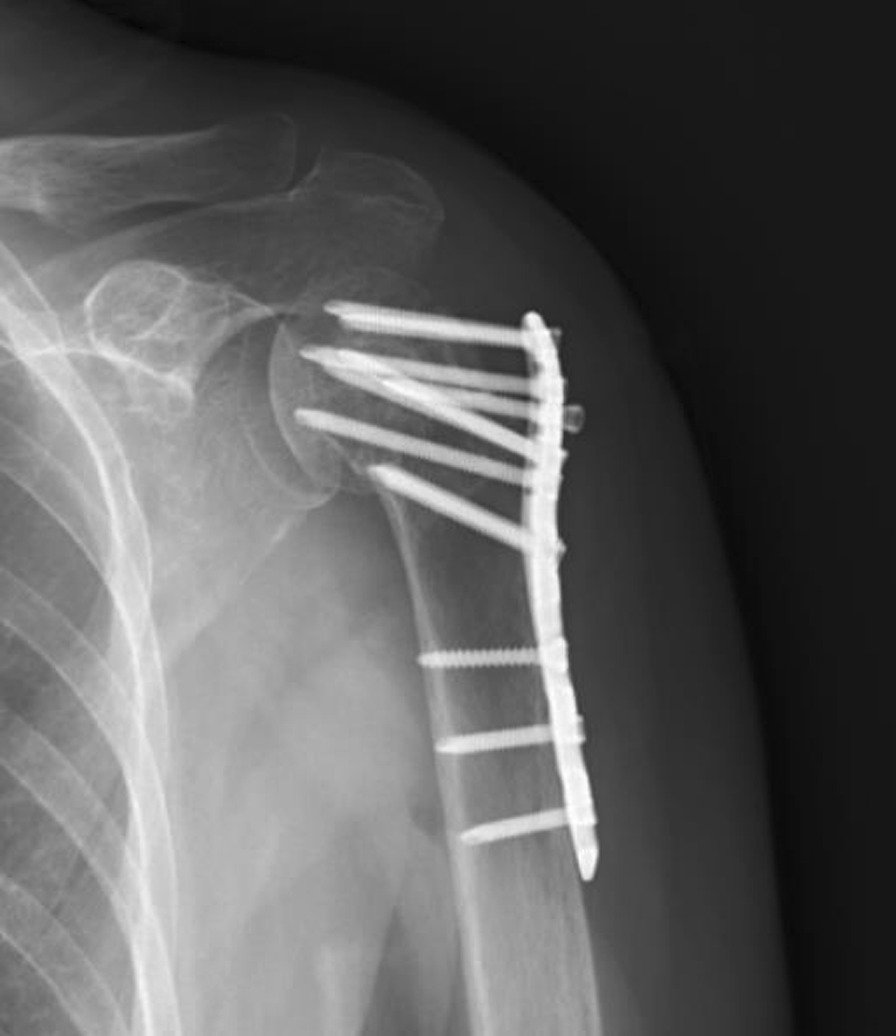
Radiograph for a patient with GT osteolysis 12 months after surgery
